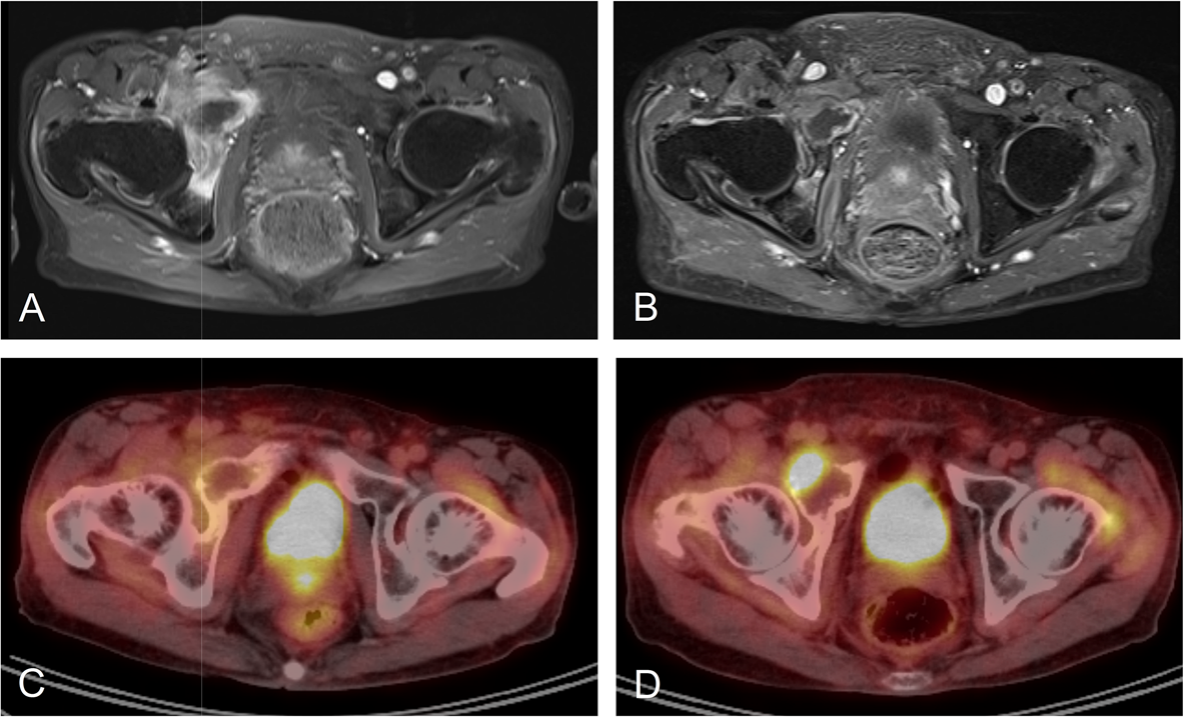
A recurrent tumor in a 79-year-old male with dedifferentiated chondrosarcoma. There were no mass lesions detected by enhanced MRI before (a) or after (b) the metastasis. CIRT induced a remission of the FDG accumulation noted by FDG-PET/CT three months after CIRT (c); however, a new lesion with high SUV appeared five months after CIRT (d)
